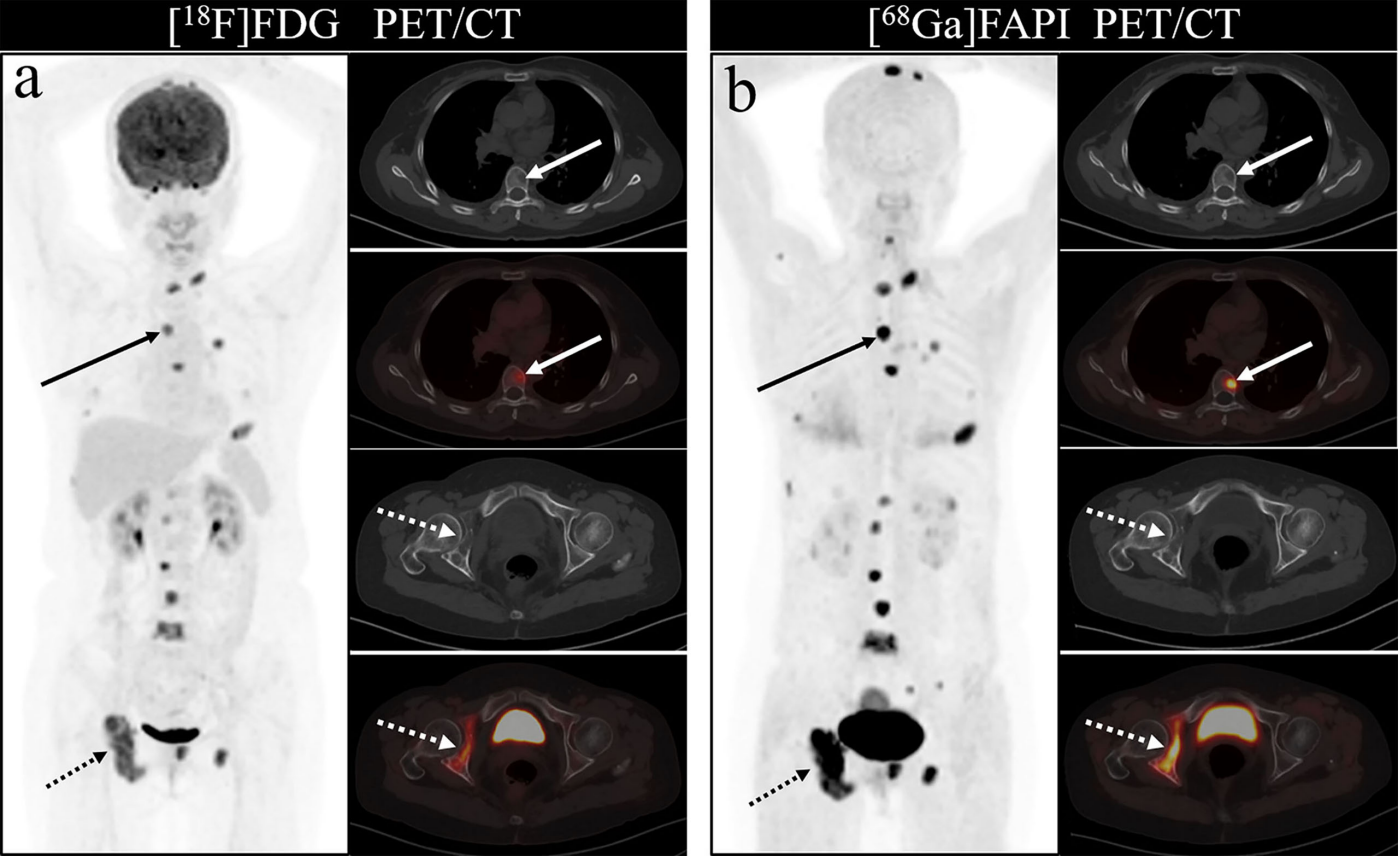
One of the patients’ [^18^F]FDG (A) and [^68^Ga]Ga-DOTA-FAPI-04 PET/CT (B) images. The figure is dapted from Wu et al. This research was originally published in Frontiers in Oncology.
